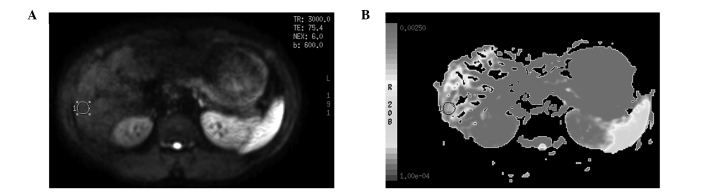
(A) Original DWI following TACE with b=600 sec/mm^2^. (B) Same patient’s pseudo-color graph of peer lever that is the same with picture A. TACE, transcatheter arterial chemoembolization; DWI, diffusion-weighted image.
